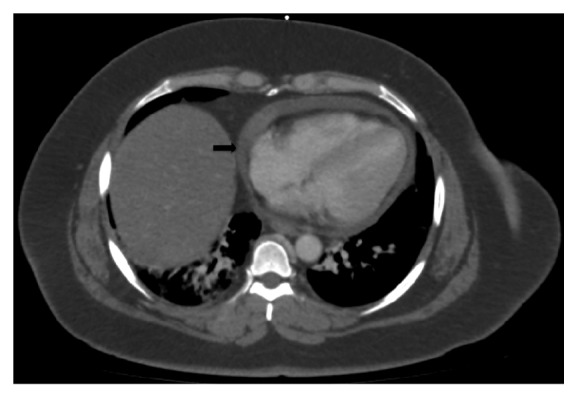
Computed tomography showing small pericardial effusion.
